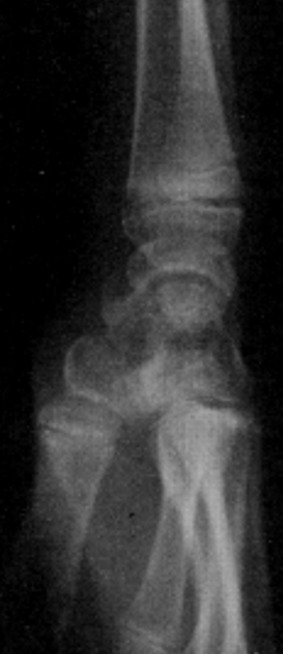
Profil radiograph shows healing of the lunate
